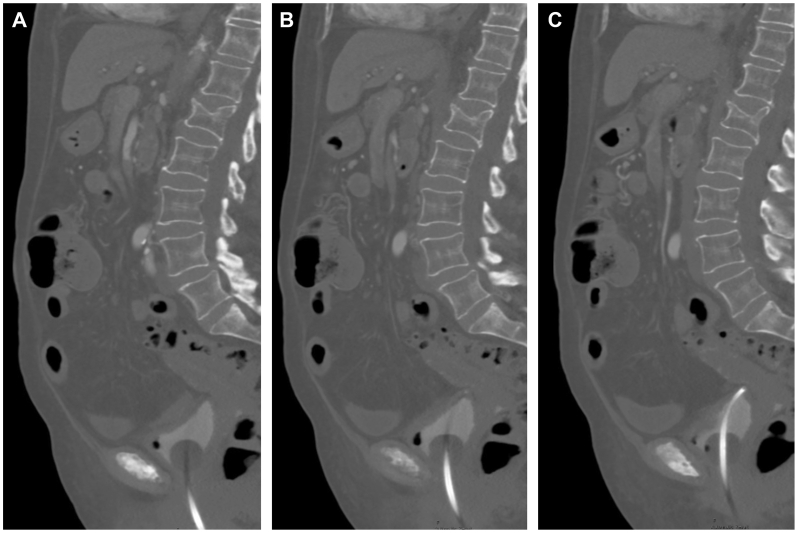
Sagittal views of computed tomography angiography (CTA) of the abdomen/pelvis demonstrating the proximal (A), mid (B), and distal (C) extents of the superior mesenteric artery (SMA) occlusion with reconstitution.
